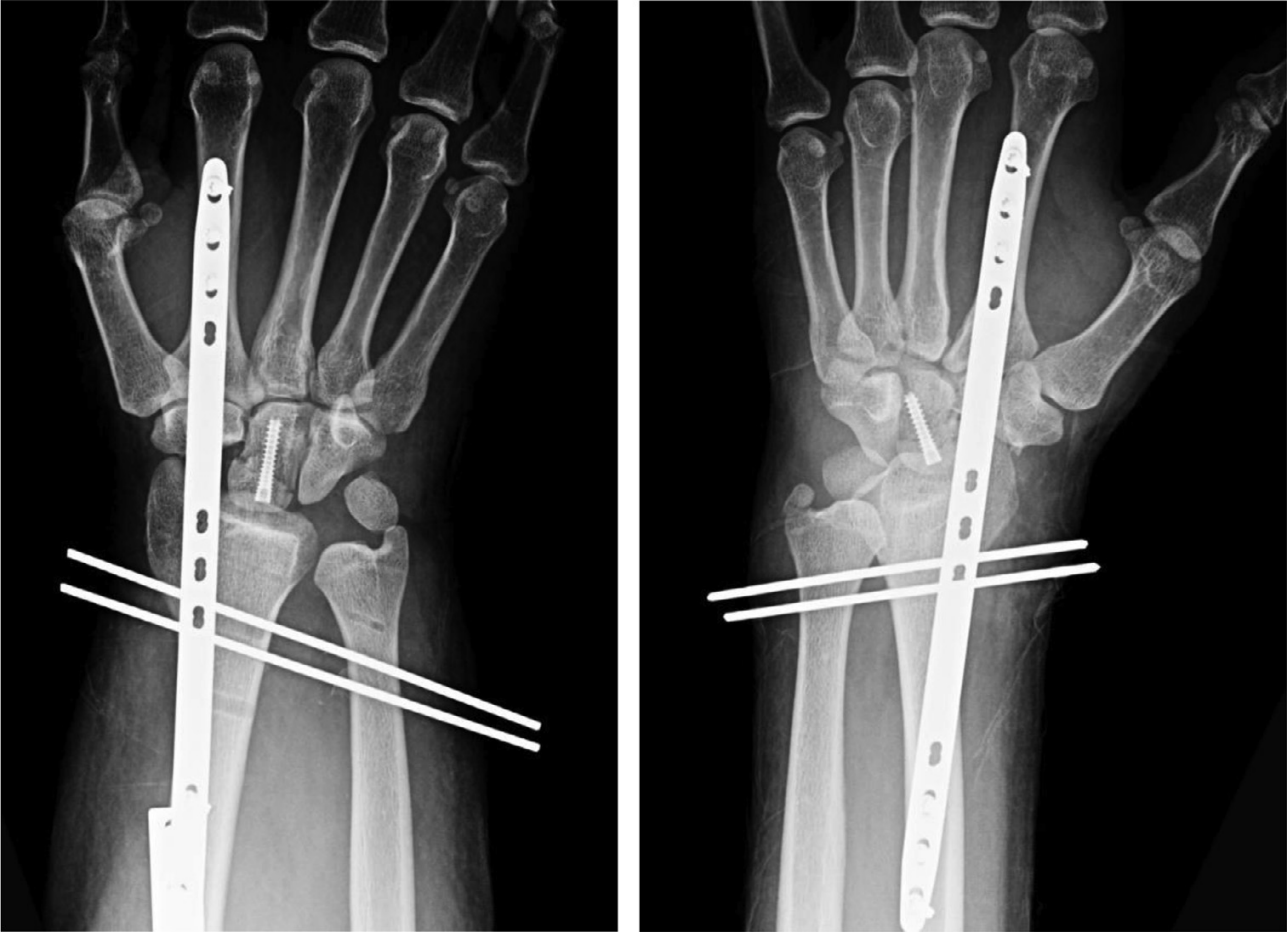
Patient 2: Postoperative radiographs of the bilateral wrists demonstrate proximal row carpectomy, fixation of the capitate with a headless screw, radiocarpal fixation with a long dorsal plate that spans the distal radius and index metacarpal, and percutaneous pinning of the DRUJ. Postoperative findings are similar bilaterally with the exception of partially imaged plate and screw fixation of the radial diaphysis on the right and a nondisplaced ulnar styloid fracture on the left.
